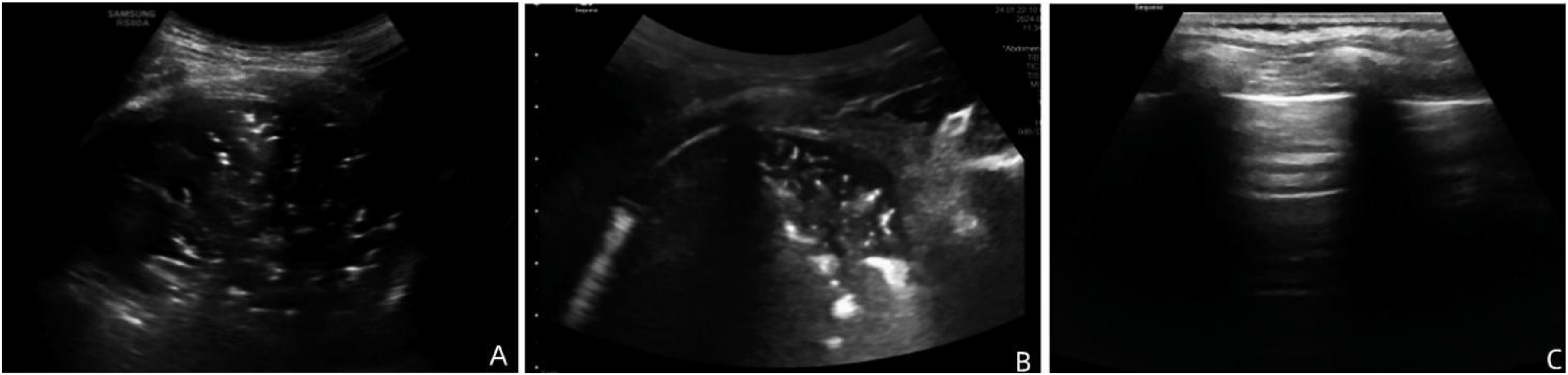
Lung ultrasound findings during the course of the disease in the pediatric patient. (A) LUS score of 25 before treatment; (B) LUS score of 13 on day 5 of treatment; (C) LUS score of 1 on day 14 of treatment.
